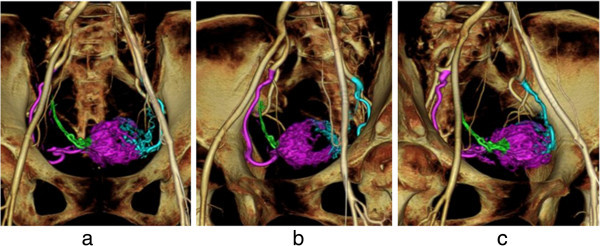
A case of uterine bleeding following surgical removal of a destructive mole. Hemorrhage-responsible arteriogram (HRA) created by CT during pelvic arteriography depicts the right (purple color) and left (blue color) uterine arteries (a). The left anterior oblique HRA image enabled easy insertion of the catheter into the right uterine artery (purple color) (b), while the right anterior oblique view enabled clear visualization of the left uterine artery (blue color) (c). Only the right uterine artery was embolized (using gelatin sponge particles) to retain the patient’s ability to bear children in the future.
